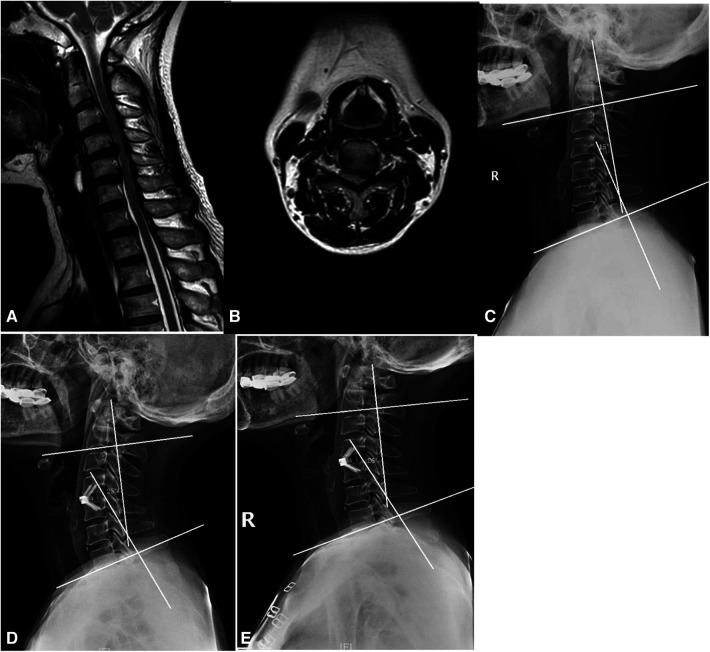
ACDF using Zero-P. (A) Sagittal MRI. (B) Axial MRI. (C) C2−7 cobb angle was 15°. (D) Immediately after surgery, C2−7 cobb angle was 26°. (E) C2−7 cobb angle was 25° at the last follow-up visit.
